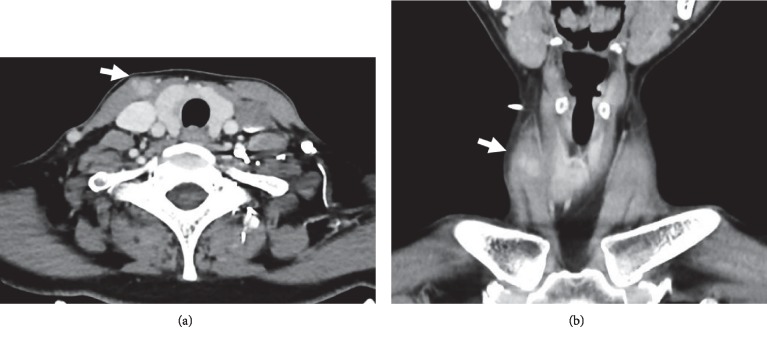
Right sternocleidomastoid muscle nodules demonstrated by white arrows on computed tomography of the neck in (a) axial view and (b) coronal view.
